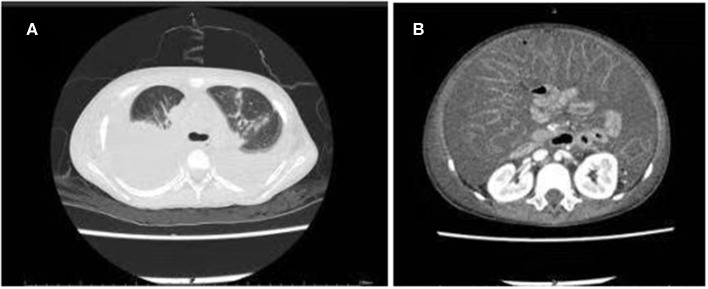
Computed tomography scan of the chest and abdomen showing multiple consolidations in both lung fields: (A) Pleural effusion and atelectasis bilaterally; (B) Massive ascites.
